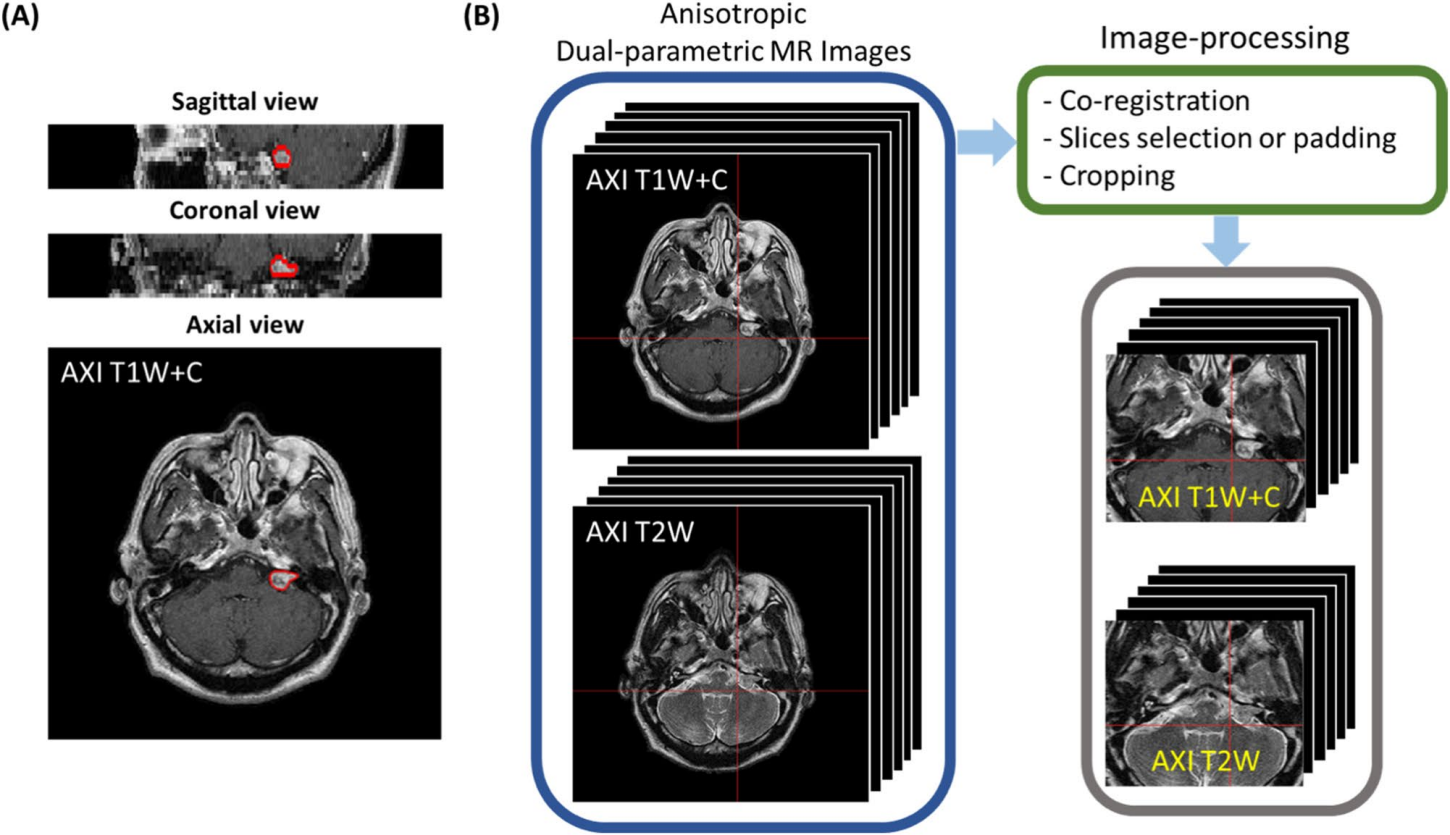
(A) Sagittal, coronal, and axial views of anisotropic MR images; (B) these raw MR images will be processed follow the steps showing here: co-registration between the various parametric images, and unified the image volume size before input our proposed dual-pathway U-Net model.
